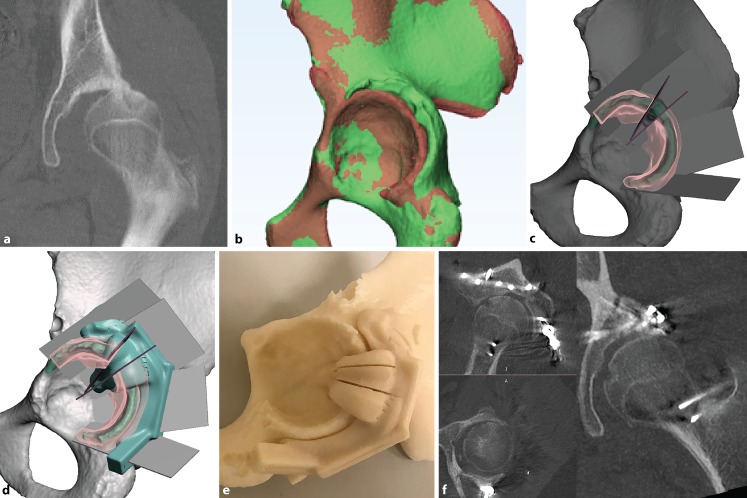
A 15-year-old patient with a malunited acetabulum posterior wall with hip subluxation (a). Digital model (*green*) compared to the mirrored opposite (*red*) (b). A closing-wedge volume-reducing osteotomy is planned (c) with 3D printed cutting jig (d, e). Postoperative computed tomography showing satisfactory restoration of hip congruency (f)
